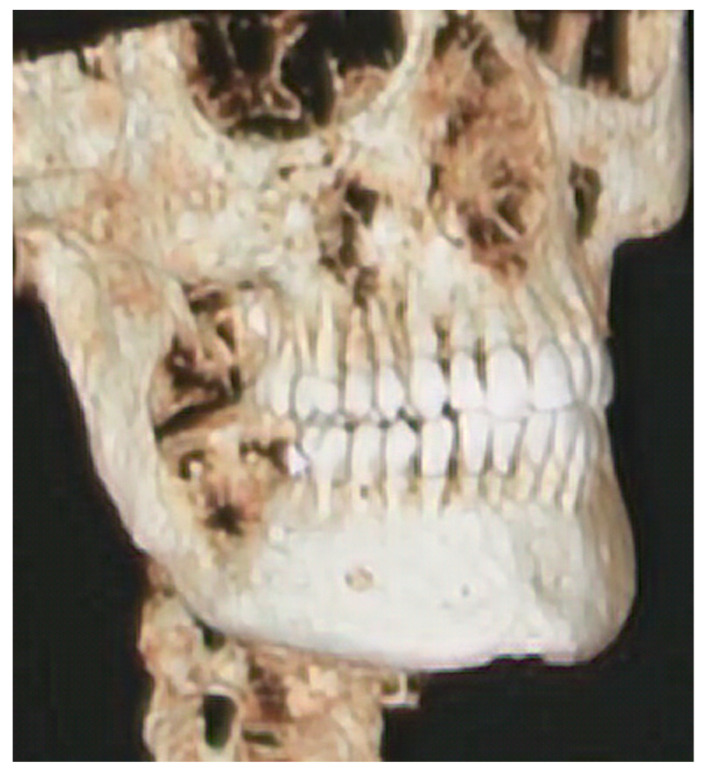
CT scan 3D reconstruction showing the ameloblastoma lesion involving the body and ramus of the right mandible.
